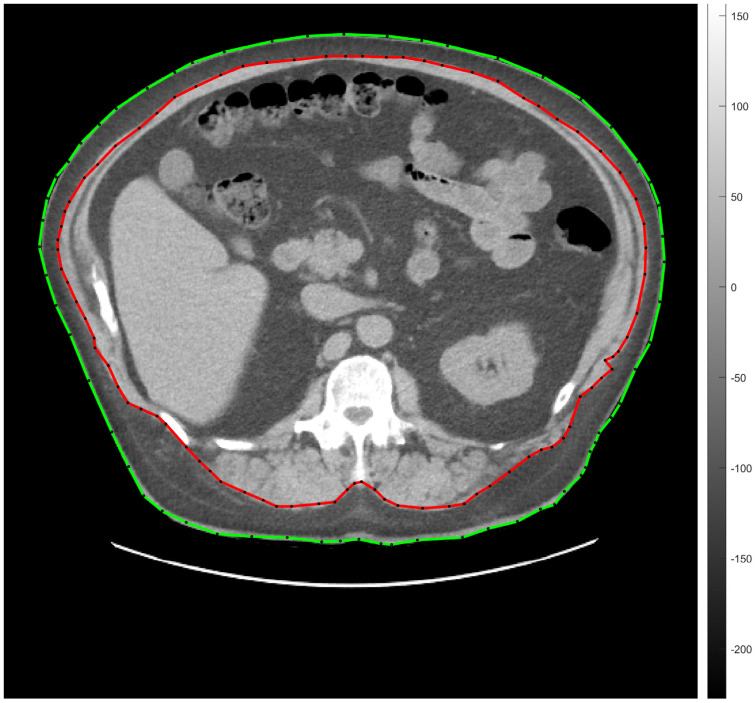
Transaxial low-dose CT slice acquired at the level of vertebra L1 for the representation of semi-automatic abdominal fat tissue segmentation. The green line indicates the body contour, the red one is for the abdominal cavity. SAT and VAT were calculated by counting the segmented pixels between the green and the red ROIs, and within the area of the red ROI, respectively. CT, computed tomography; L1, vertebra lumbar I; SAT, subcutaneous adipose tissue; ROI, region of interest; VAT, visceral adipose tissue.
